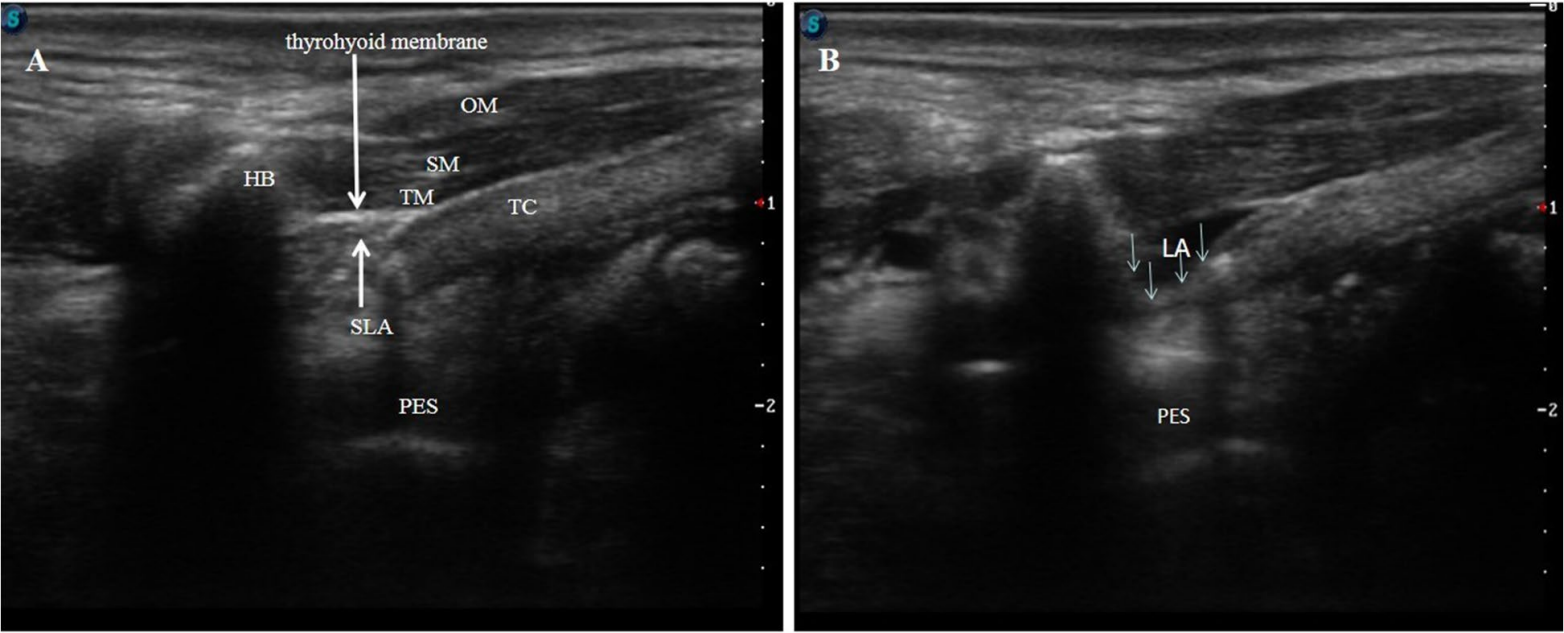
A Ultrasound images of a longitudinal iSLN. B Local anesthetic was injected into the superior laryngeal nerve space. OM, omohyoid muscle; SM, sternohyoid muscle; TM, thyrohyoid muscle; SLA, superior laryngeal artery; LA, local anesthetic; PES, pre-epiglottis space. TC: thyroid cartilage
